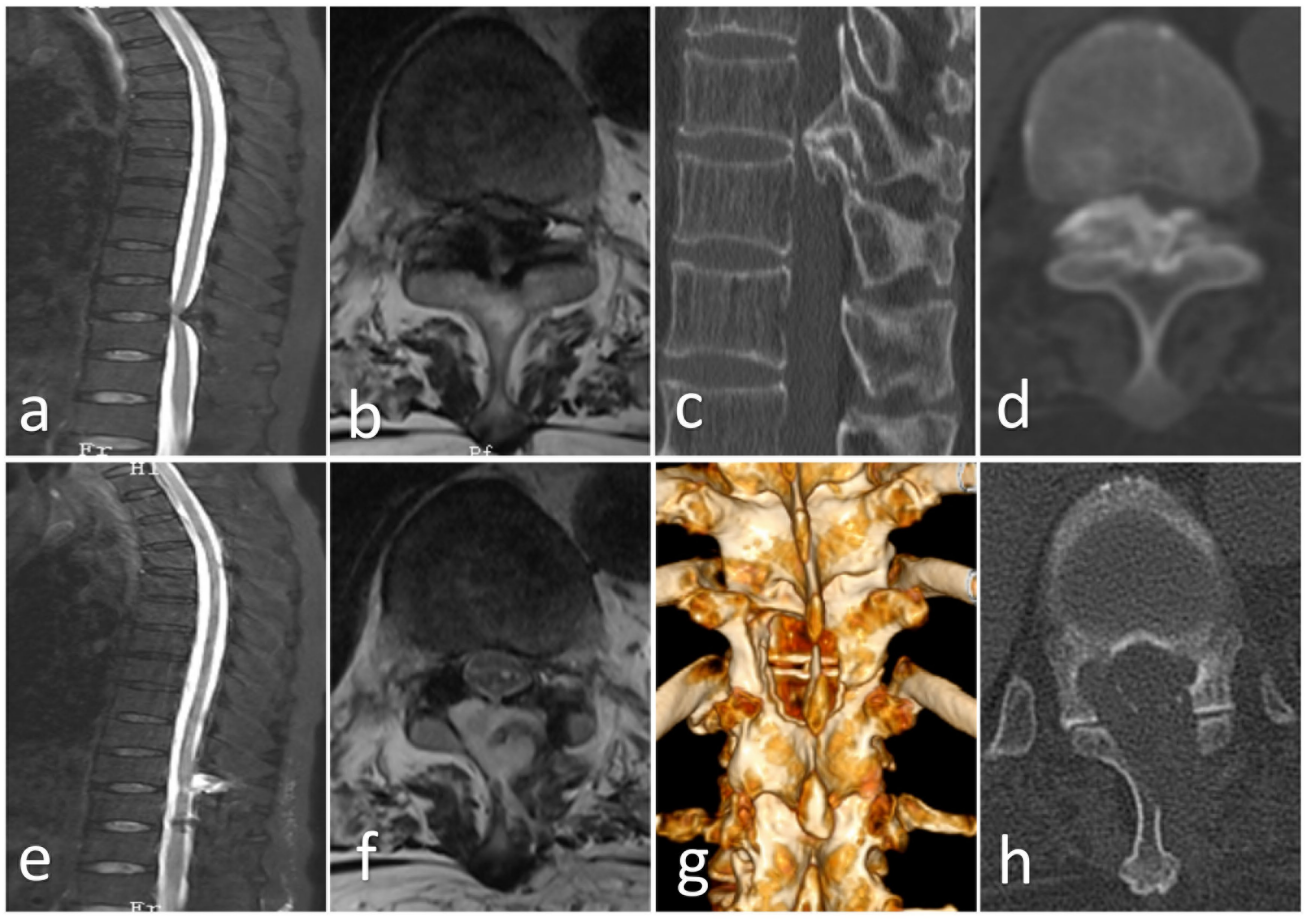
(a,b) Preoperative MRI shows ossification of the thoracic ligamentum flavum (Round); (c,d) Preoperative CT shows severe ossification of the thoracic ligamentum flavum (Fused); (e,f) Postoperative CT shows that the ossification has been removed and spinal cord decompression has been successful, and a small amount of cerebrospinal fluid leaked out; (g,h) Postoperative CT and 3D reconstruction show that the spinal canal has been opened and the ossification has been removed.
